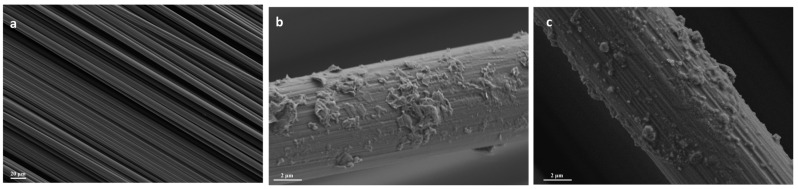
SEM images of (a) pristine carbon fiber, (b) fiber coated with 20 mgsm GNPs only, and (c) fiber coated with HBPUD/GNP-1:1 with 20 mgsm GNP deposition by ultrasonic spray deposition.
